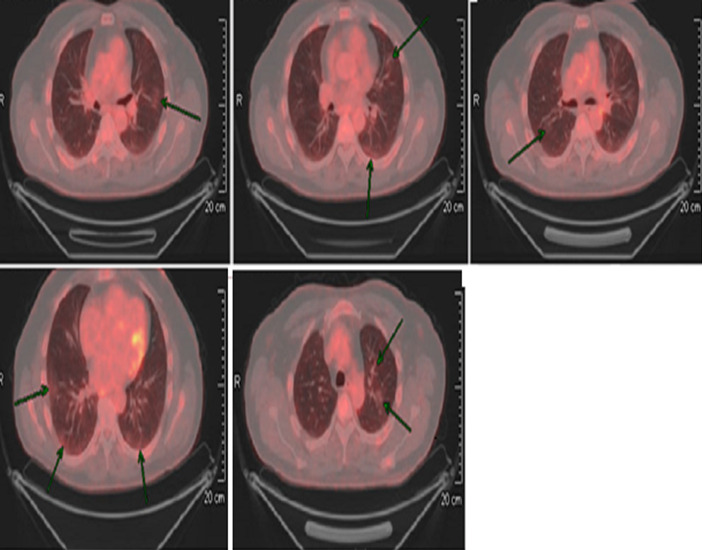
CT PET showing faintly FDG-avid patchy prominently peripheral ground-glass changes in bilateral upper and lower lobes in keeping with probable COVID-19 infection
